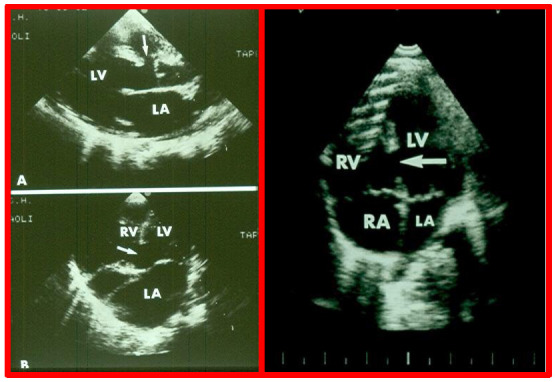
Echocardiographic visualization of VSDs. Selected video frames
